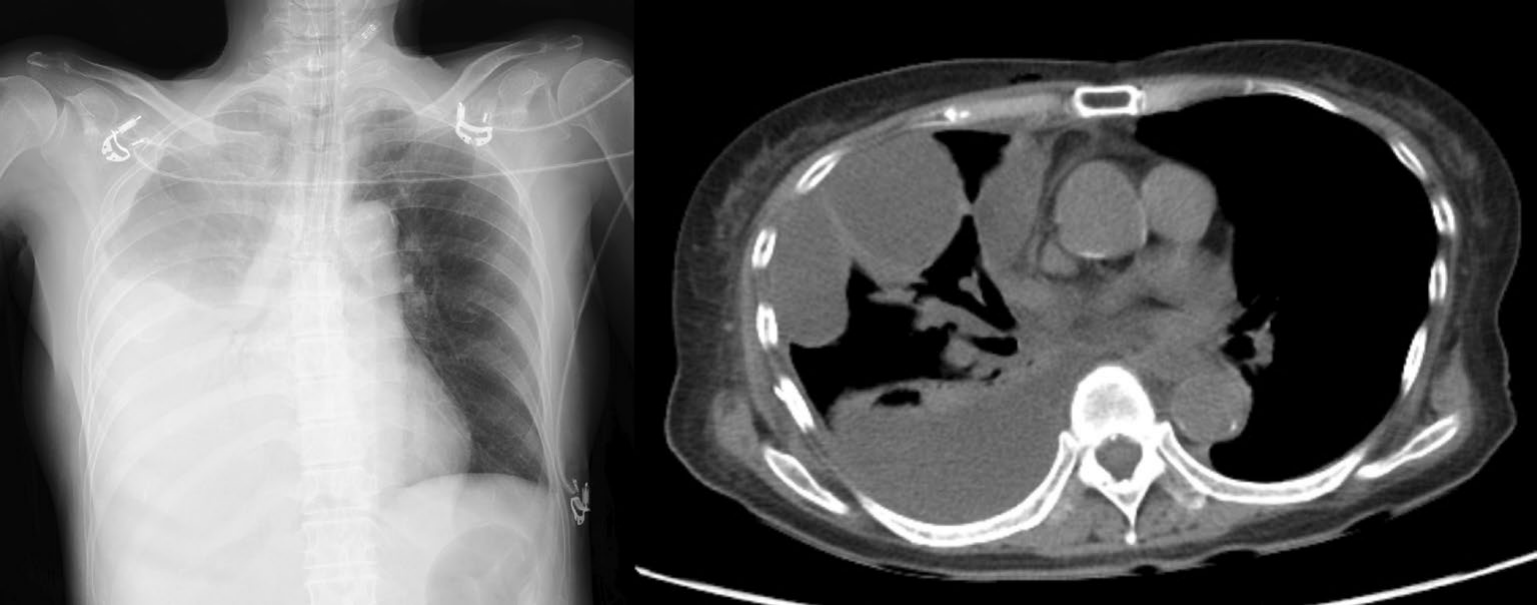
Case 2. Postoperative chest radiograph after palliative gastrointestinal bypass surgery showing a marked increase in right pleural effusion. During subsequent follow‐up, the right pleural effusion did not improve, and computed tomography confirmed multiloculated effusion.
